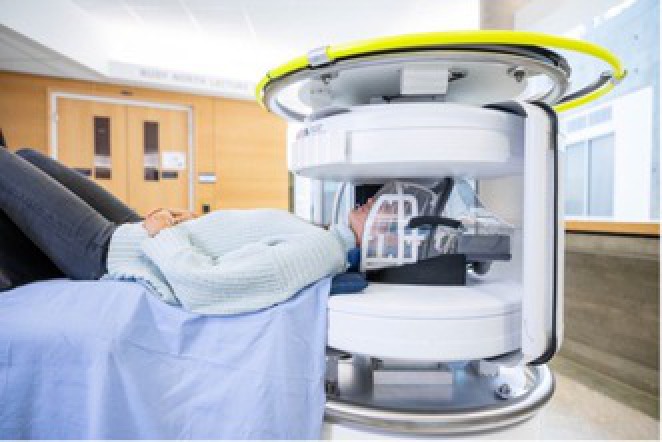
Hyperfine Swoop Ultra Low Field 0.064T MRI.
